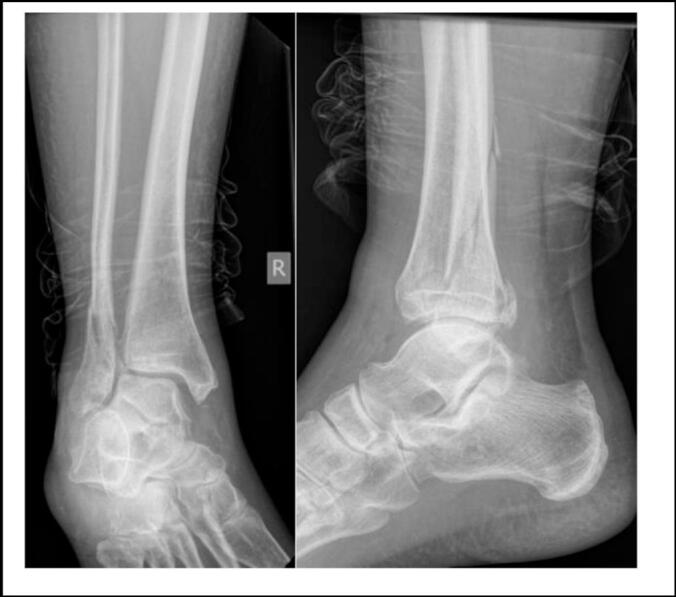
Initial AP and lateral ankle radiographs.
